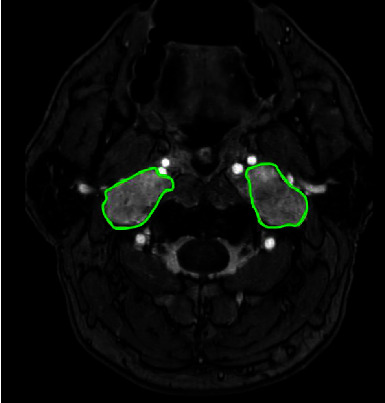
3D TOF with gadolinium MRI showing bilateral vagal paragangliomas (green outlines).
